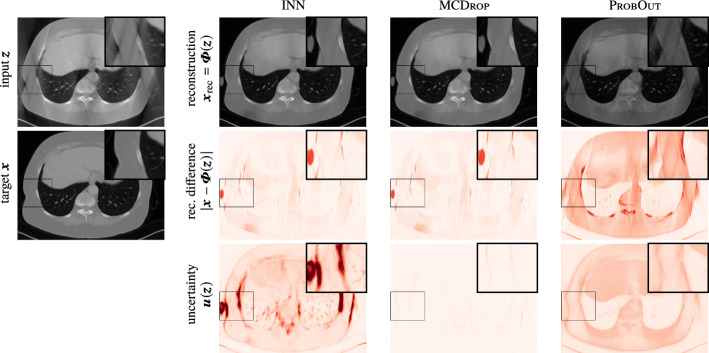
Results of three UQ methods for the Error Detection experiment for one exemplary data sample of the limited angle CT task. The plotting windows are equally adjusted for better contrast.
